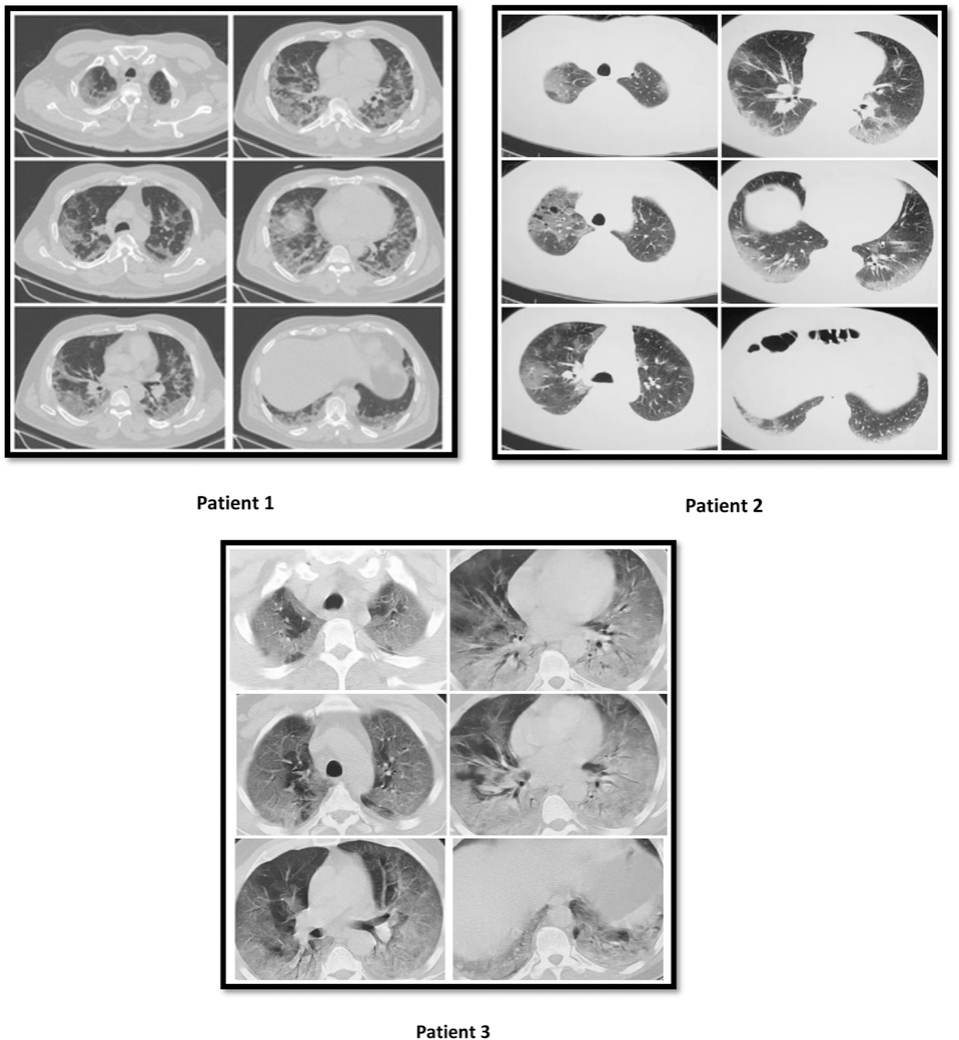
Chest computed tomography scans of patients 1, 2, and 3. All scans show diffuse ground glass opacities (GGOs), with peripheral distribution, consistent with novel coronavirus disease (COVID-19) lung involvement. Patients 1 and 2: crazy paving appearance, suggestive of late COVID-19. Patient 3: diffuse widespread GGOs and bilateral diffuse opacification due to acute respiratory distress syndrome, a severe complication of COVID-19.
